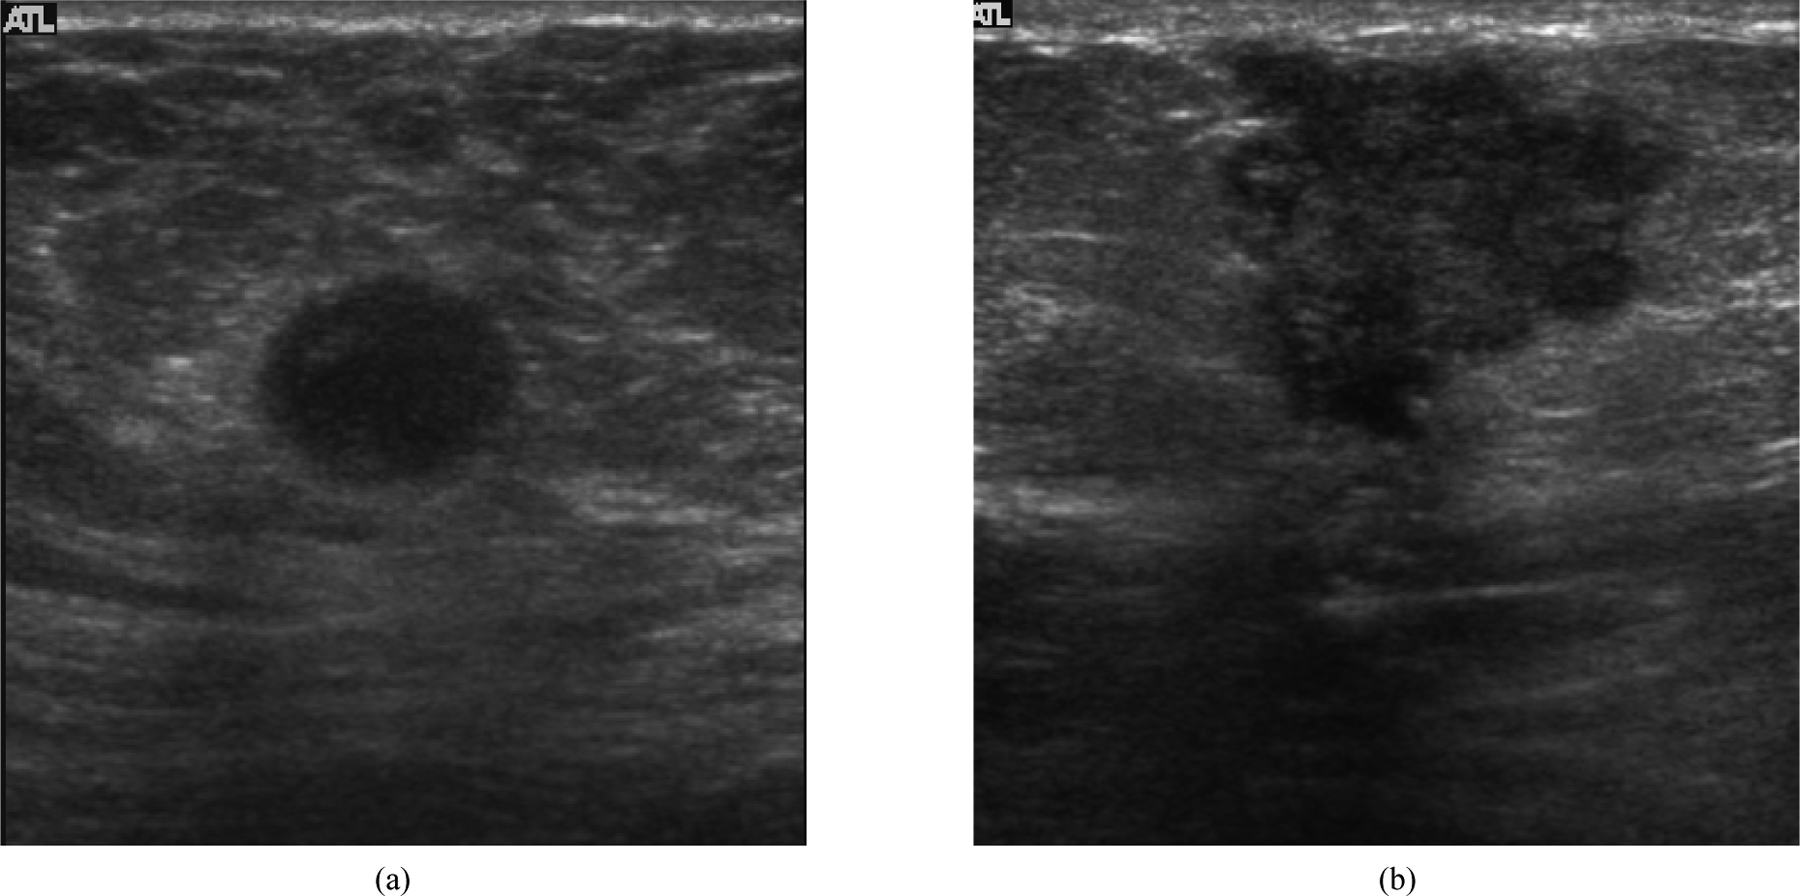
(a) Example of a breast mass that showed high agreement in sonographic features selected between the three observations; (b) Example of a breast lesion that showed lowest agreement in features selected over the three observations.
